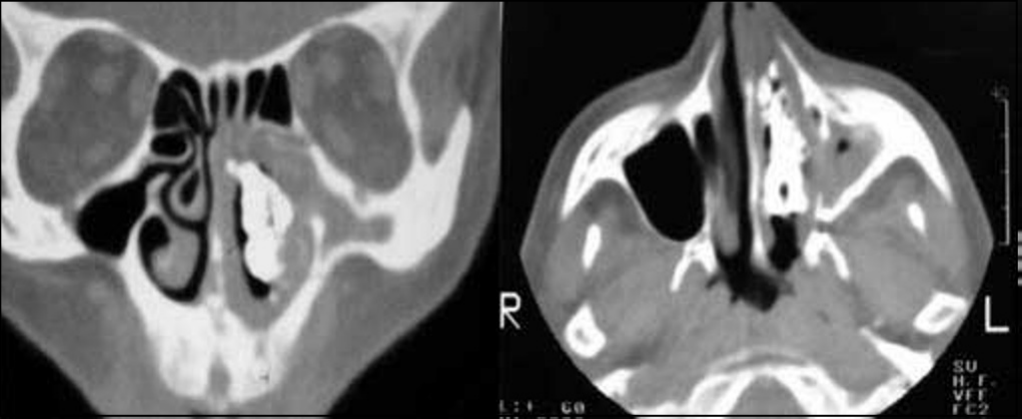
Coronal and axial CT scan sections showing large rhinolith in the left nasal fossa.
